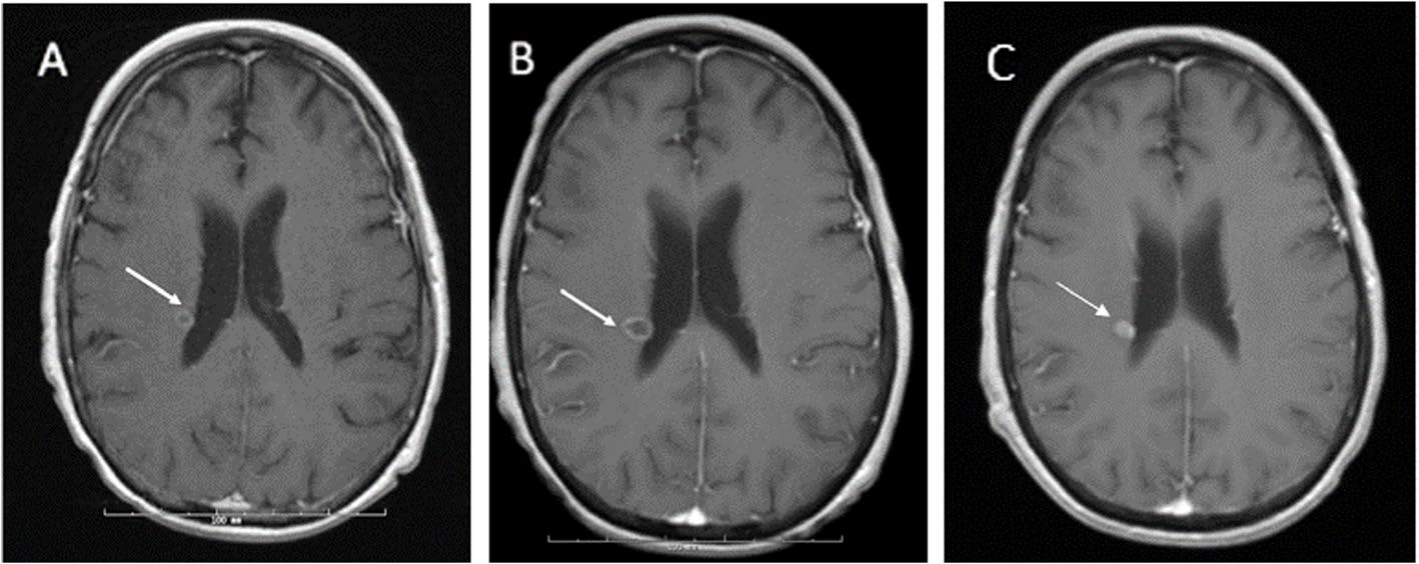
MRI brain. T1 post-contrast imaging with rim-enhancing lesion in periventricular right frontoparietal region, increased from 7 × 4 mm on day + 26 (A) to 10 × 7 mm on day + 33 (B). After 8 weeks of therapy the lesion decreased back to 7 × 6 mm on day + 83 (C)
